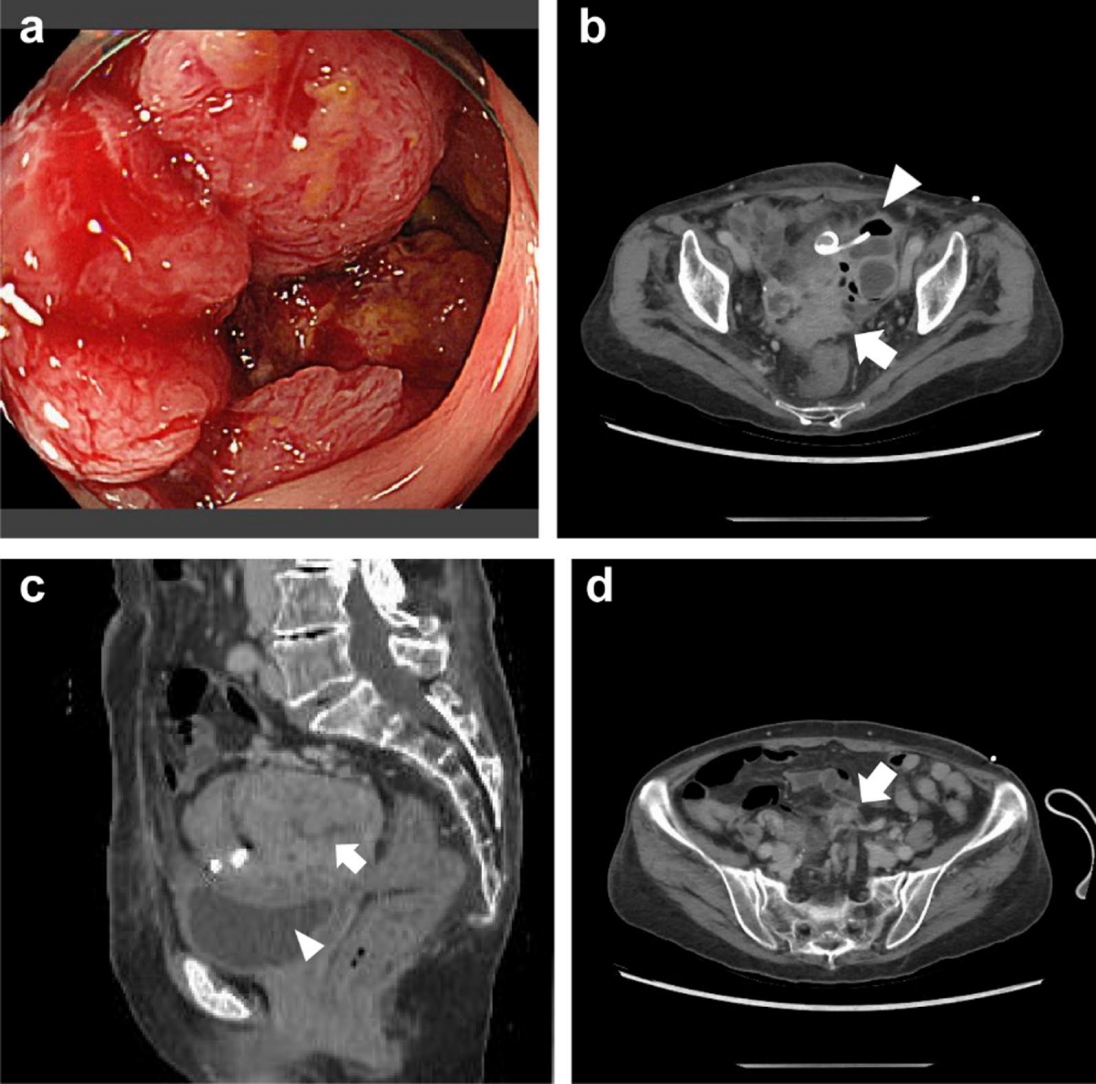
Colonoscopy and re-evaluation CT after percutaneous drainage. Colonoscopy revealed circumferential advanced cancer in the sigmoid colon (a). CT revealed a bulky tumor possibly invading the adjacent organs (arrow), and the drainage tube with residual abscess (arrowhead) (b). The fat layer between the tumor (arrow) and the bladder (arrowhead) was disappeared (c). Enlarged regional lymph nodes were also observed (arrow) (d)
